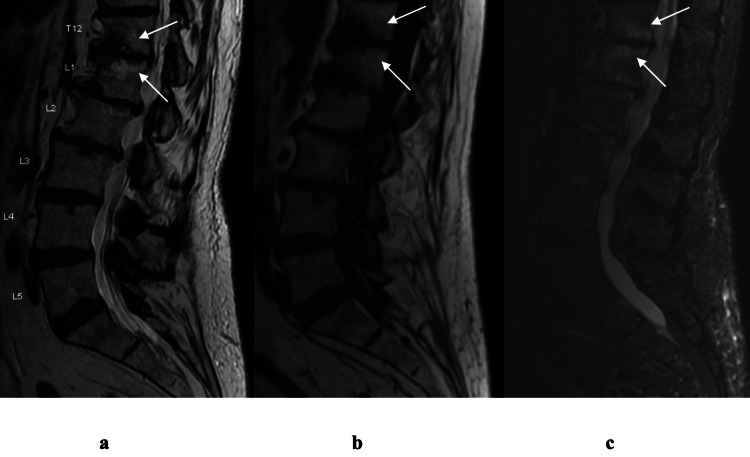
T2-weighted (a), T1-weighted (b), and STIR (c) MR images of a patient with degenerative end-plate changes with inflammation (Modic type I changes) at the T12–L1 level (white arrows in a, b, and c).
